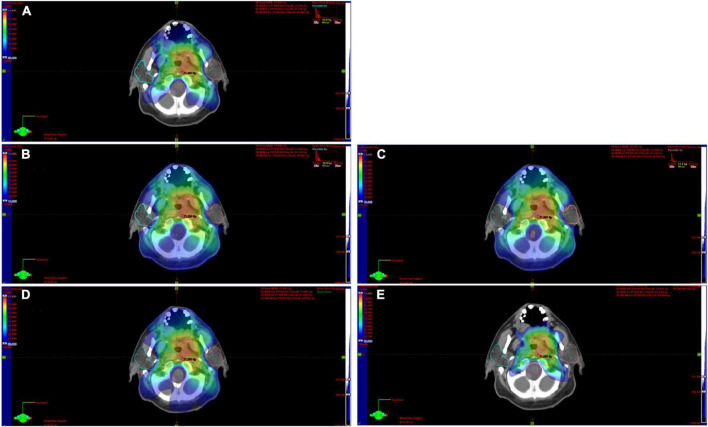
Contouring of the parotids on CT images and the related dose map. In this explanatory case, both the left and right parotid showed a D20 equal to 26.8 Gy (A). The D40 of the right parotid was equal to 14.88 (B) the left one was 15.62 (C). Panels (D,E) show the volume covered by an isodose of 20 and 40 Gy, respectively.
